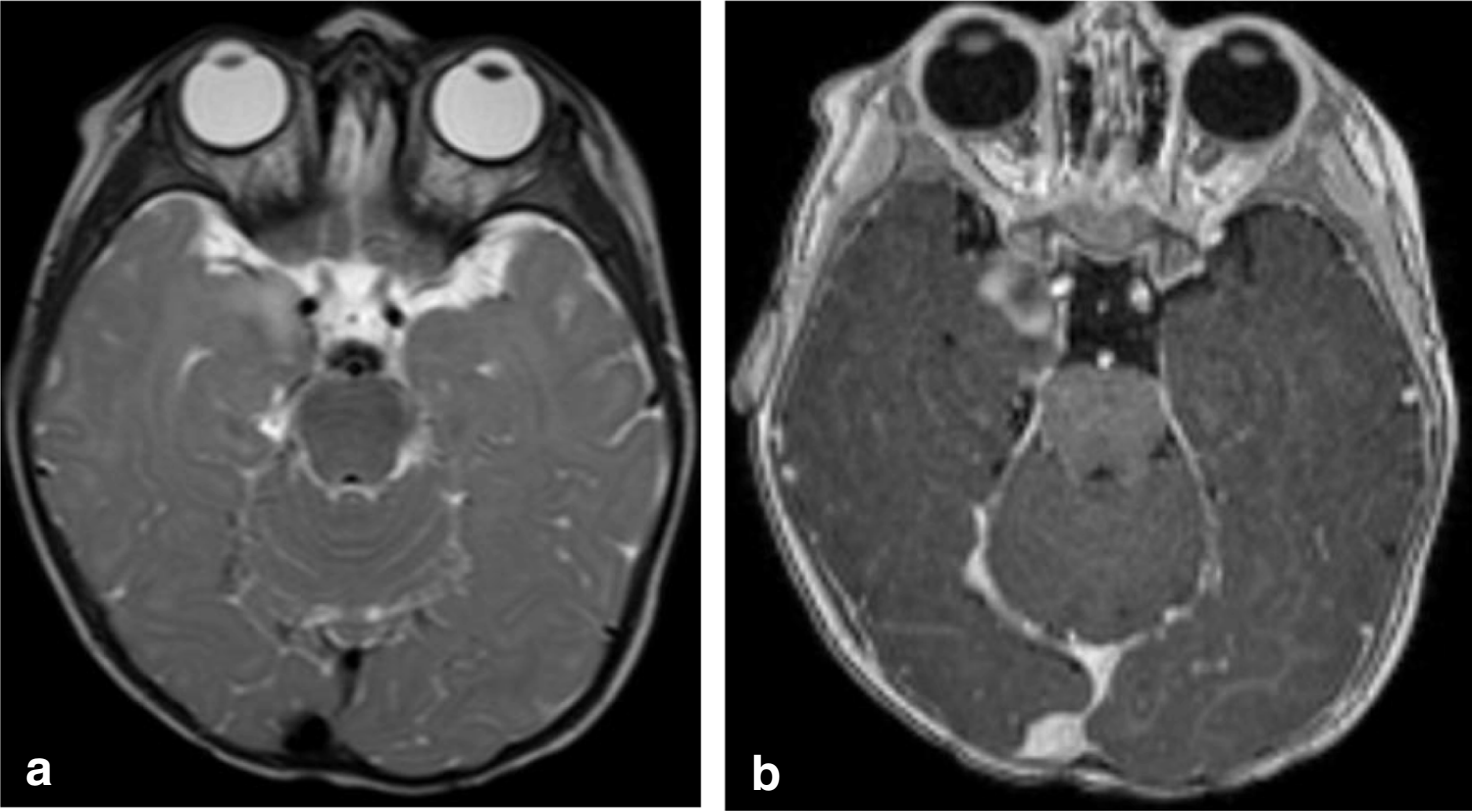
Ganglioglioma with *BRAF V600E* mutation in a 7-month-old male presenting with seizures. Axial T2-weighted (a) and T1-weighted contrast-enhanced (b) MR images show an ill-defined, mildly expansile, cortically-based right temporal lobe mass with nodular enhancement
